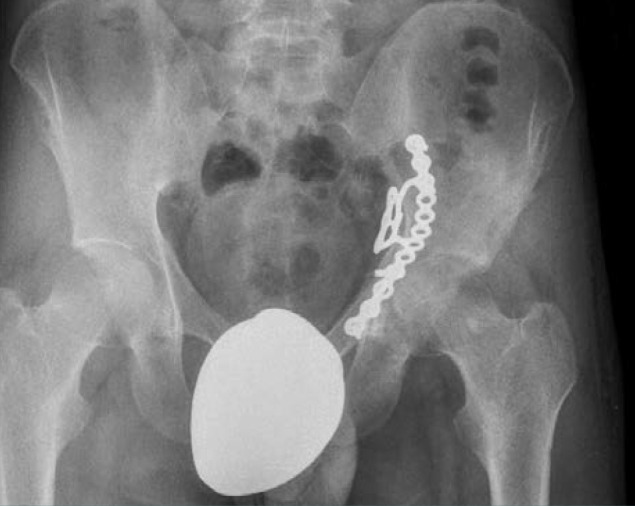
X-ray result after 6 months
